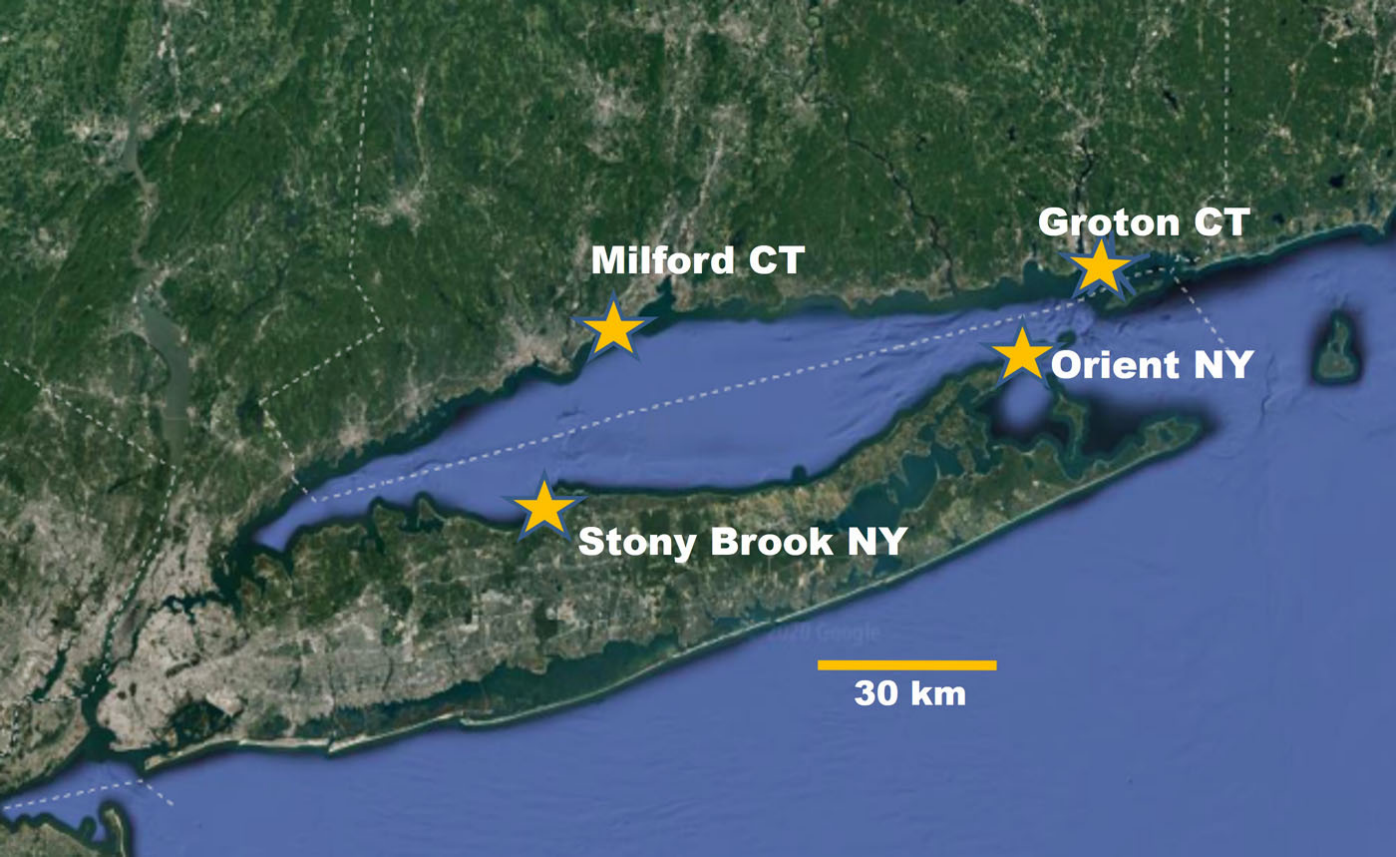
Map of Long Island Sound and sites where blue mussels were collected (Google Earth image). Milford CT, 41°12′35″N 73°03′03″W; Groton CT, 41°19′04″N 72°03′51″W, Orient NY 41°09′34″N 72°14′05″W; Stony Brook NY, 40°55′16″N 73°09′00″W.
